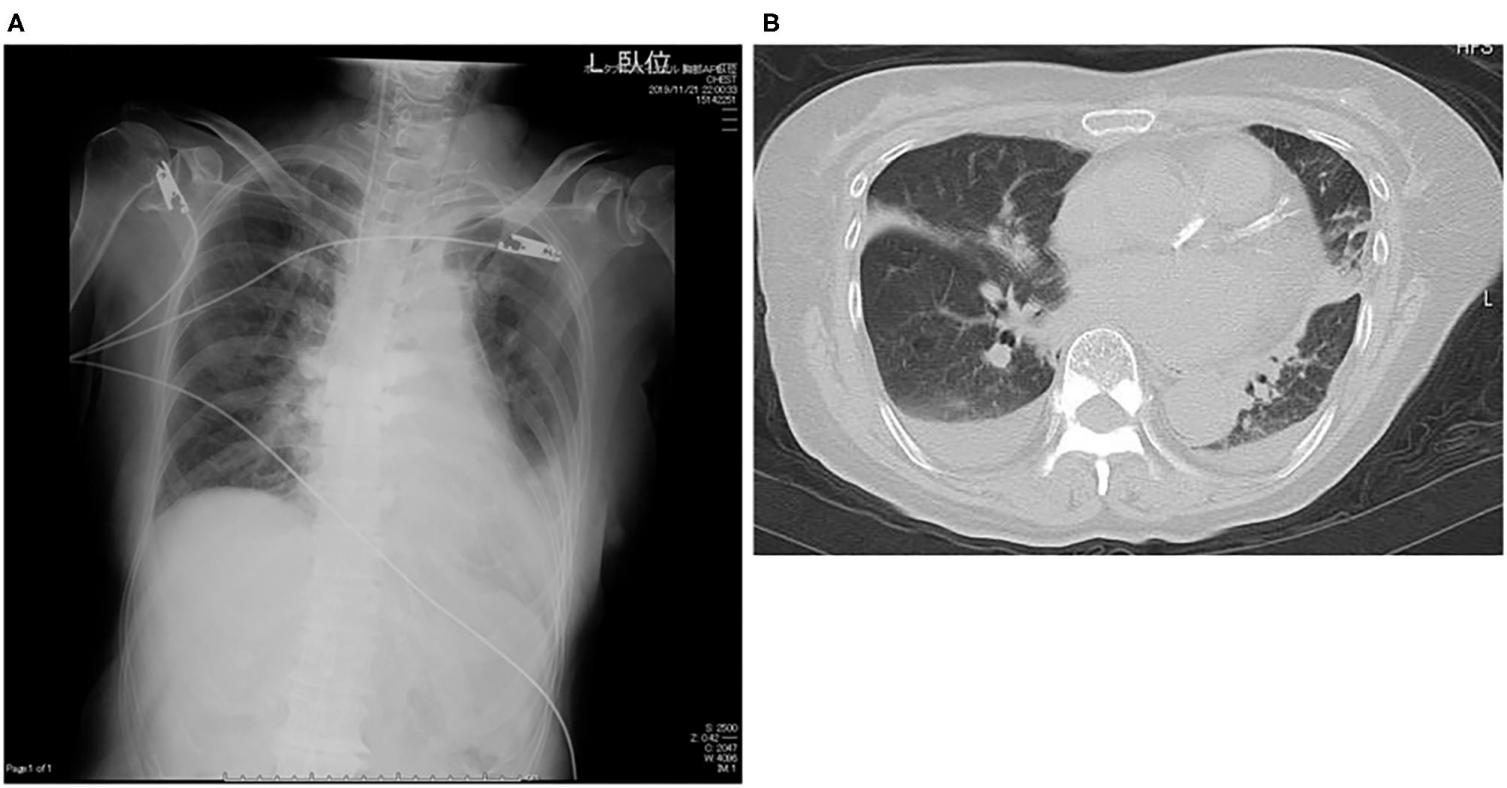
Chest X-ray on admission (A) and chest computed tomography during hospitalization (B).
